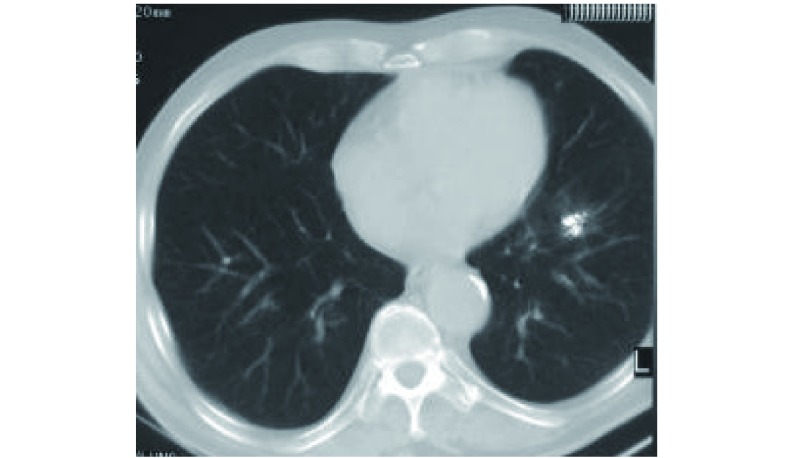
术后6个月胸部CT。胸部CT可见肿瘤较术前缩小60%以上，肿瘤周围出现轻度放射性肺炎改变。 The chest CT scan of 6 months postoperative. This chest CT scan shows the tumor reduced more than 60%, and there is slight change of radiational pneumonitis around it.
